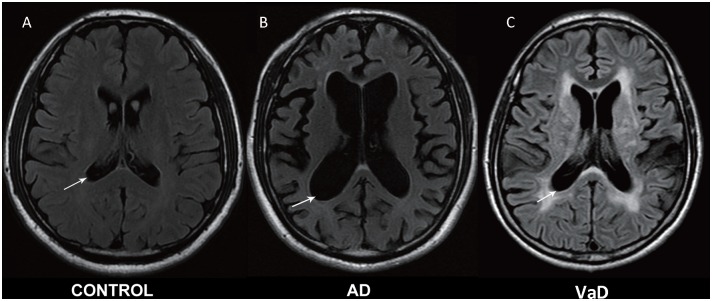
Magnetic resonance imaging (MRI) images in normal control, Alzheimer’s disease (AD) and vascular dementia (VaD) patients. (A) Healthy control. (B) Cerebral atrophy and enlargement of the cerebral lateral ventricles of AD patients. (C) Leukoencephalopathy and encephalomalacia foci of VaD patients. All MRI are shown in T2-weighted image.
